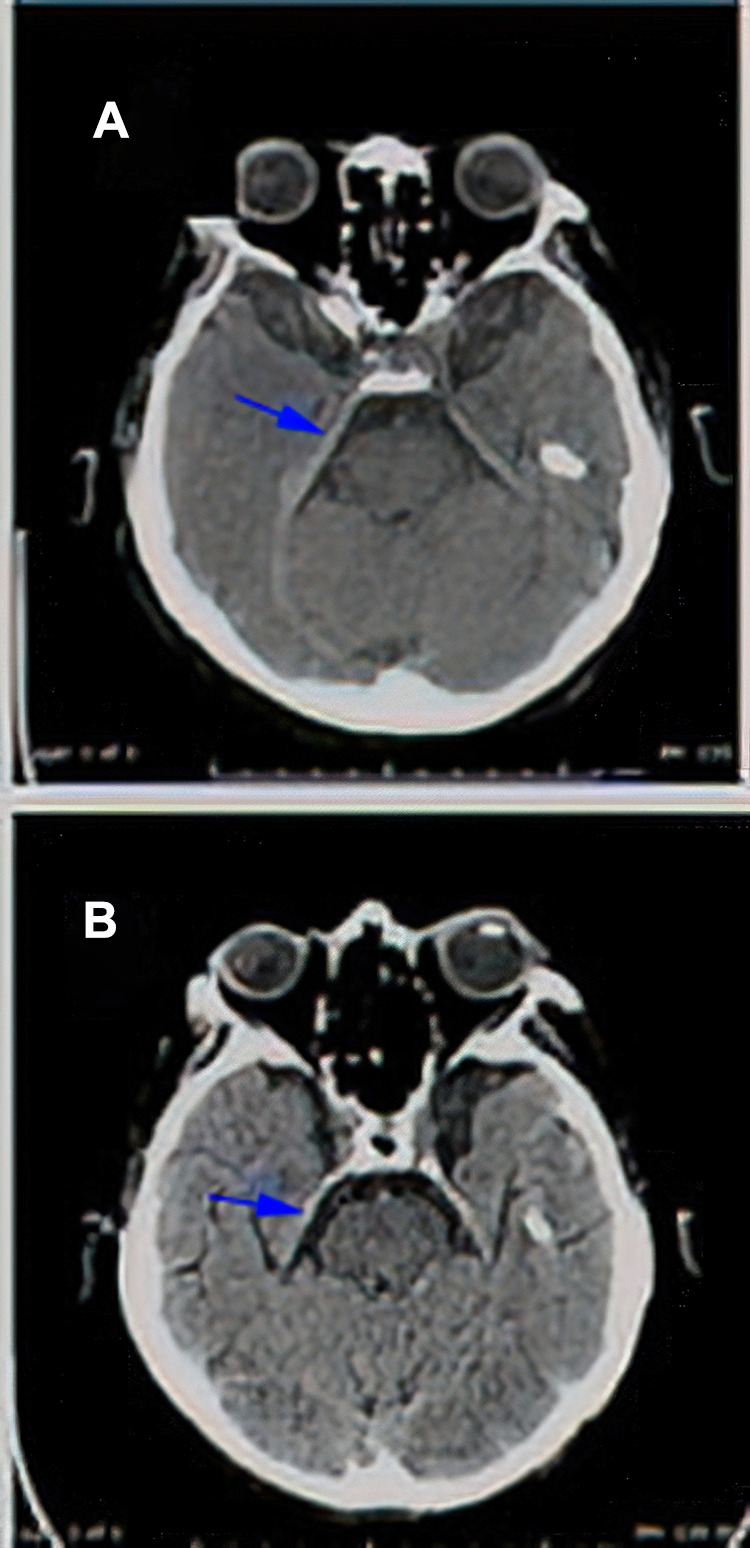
(A) Axial CT scan of the brain without IV contrast (patient cannot do MRI and cannot receive IV contrast because of rising creatine), this limited study shows thickening of the right tentorium in comparison to left one suggesting meningeal involvement (arrow in A). (B) Axial CT scan of the brain without IV contrast (patient cannot do MRI and cannot receive IV contrast because of rising creatinine), shows almost complete clearance of the thickened right tentorial leaflet (arrow in B).
